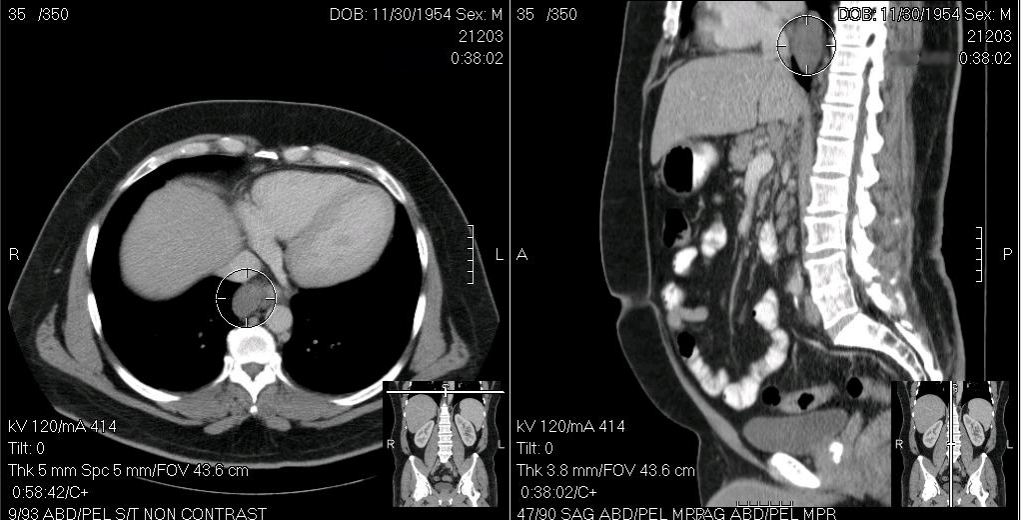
CT scan images of the posterior mediastinal mass.
